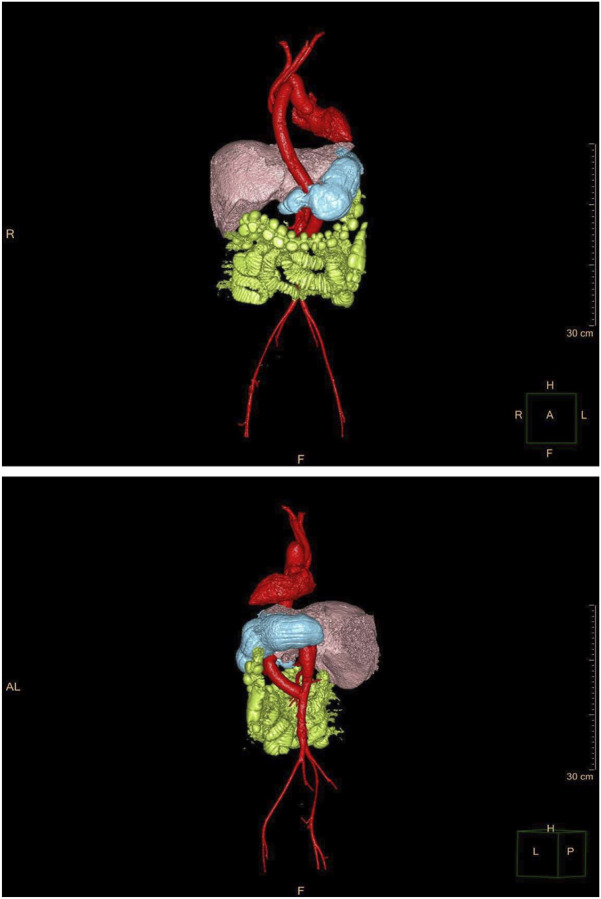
3D CT reconstruction images demonstrating the anatomical position of the extra-anatomic bypass graft (red) relative to adjacent organs (liver: pink; stomach: blue; intestine: green) after the first-stage procedure.
